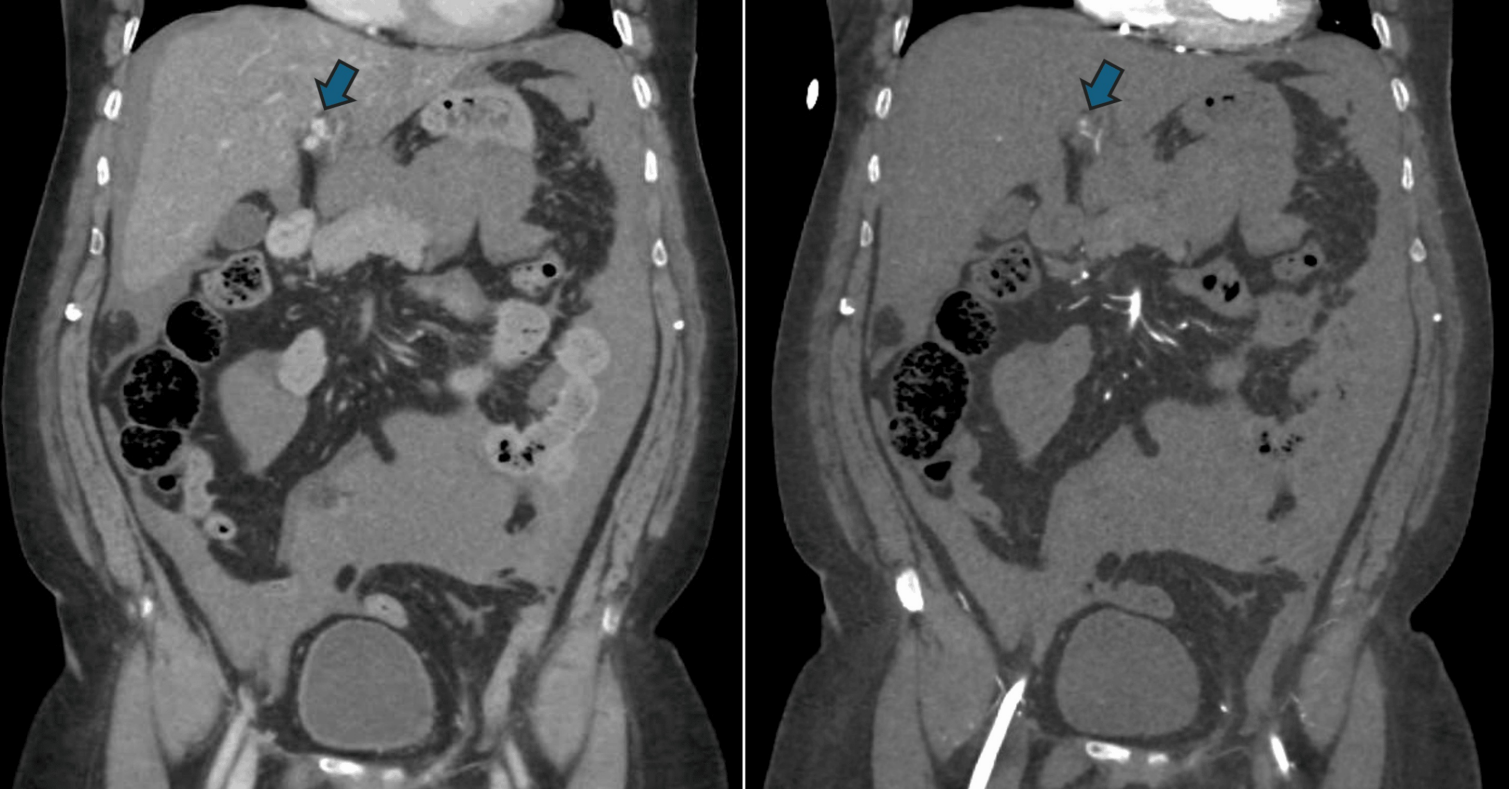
CT scans in the portal venous phase (left) and the arterial phase (right) showing hemoperitoneum and abnormal dilatation of a branch of the left hepatic artery with contrast extravasation (arrows).
